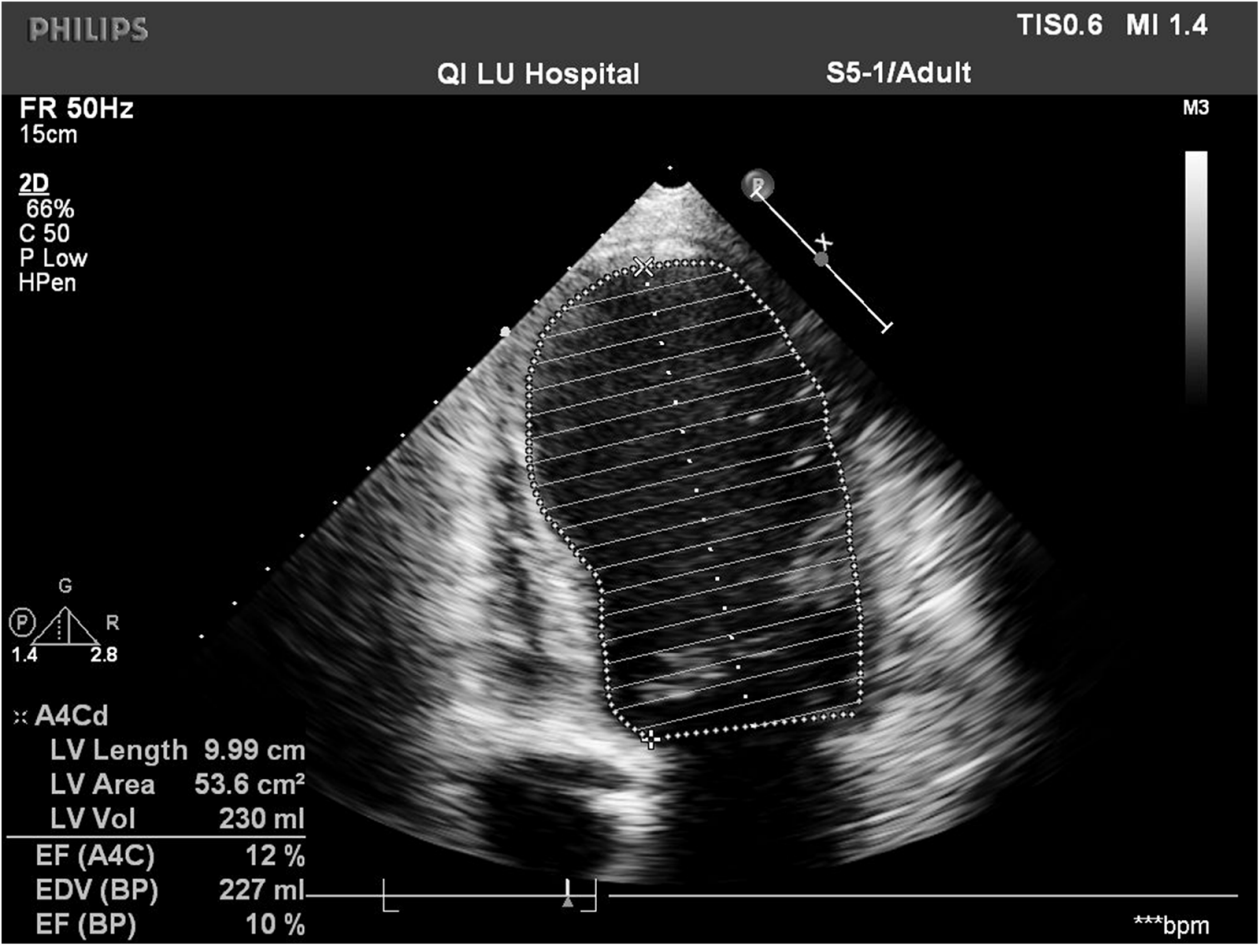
Bedside cardiac ultrasound showed significantly low left ventricular ejection function (LVEF 10%).
